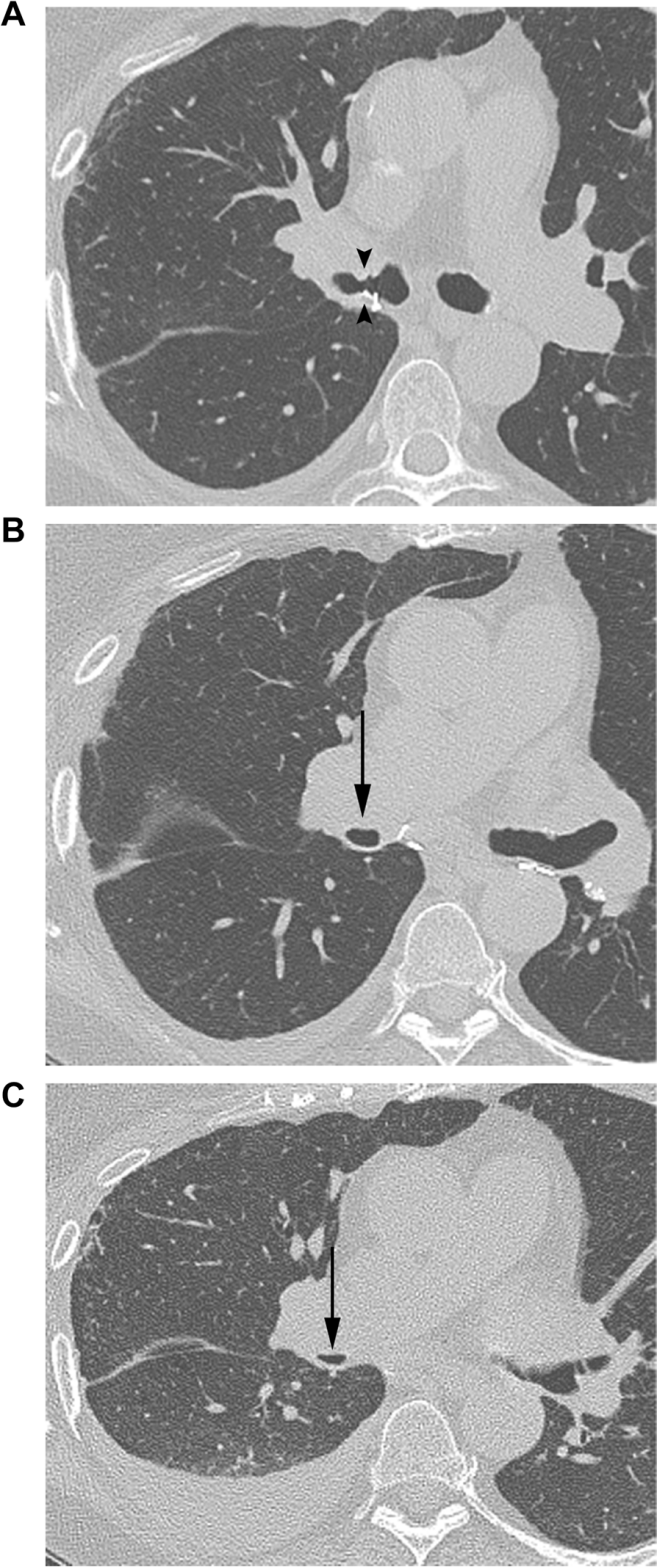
67-year-old male who underwent bilateral lung transplants for COPD with bronchial stenosis and bronchomalacia. A Axial CT image (lung window) shows right sided bronchial anastomotic stenosis (arrowheads), previously discovered on bronchoscopy. B, C Inspiratory (B) and expiratory (C) axial CT images in lung window show a patent bronchus intermedius (arrow in B) with collapse of the anterior cartilaginous portion on expiratory phase (arrow in C) suggesting bronchomalacia
